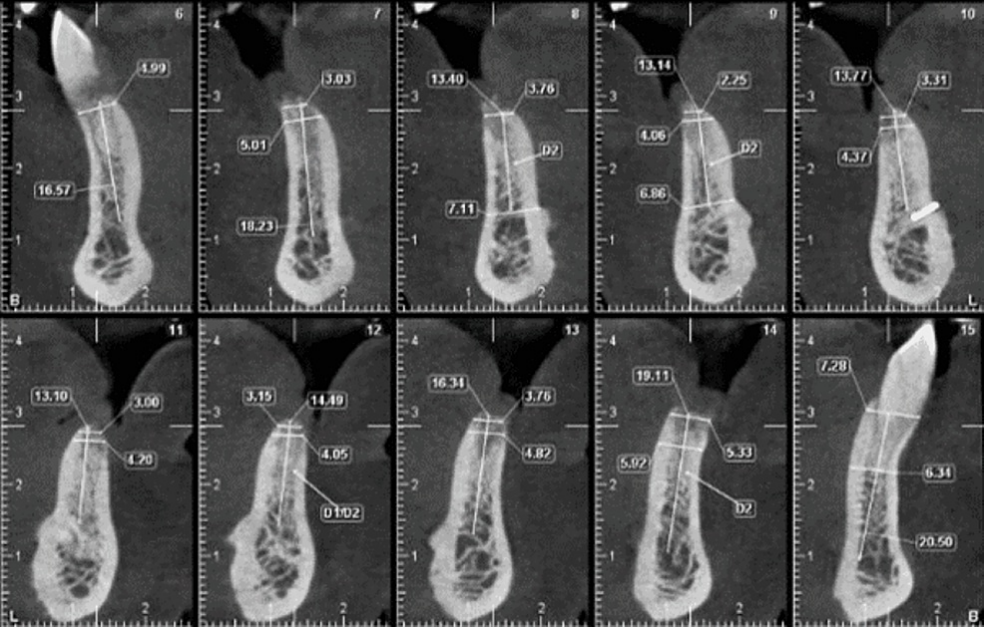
Preoperative CBCT of the anterior mandible showing the bone width at different sections. CBCT, cone beam computed tomography
